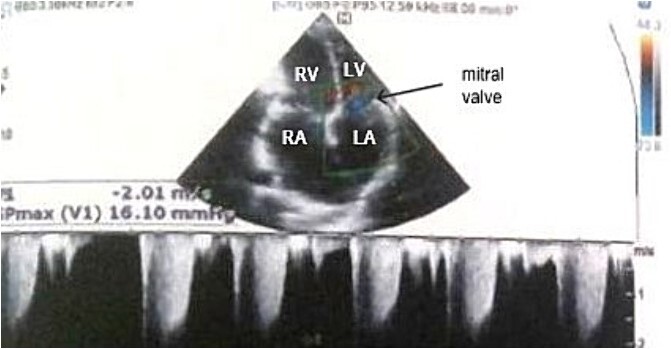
Transthoracic echocardiography showed moderate mitral regurgitation. RA: right atrium, RV: right ventricle, LA: left atrium, LV: left ventricle.
